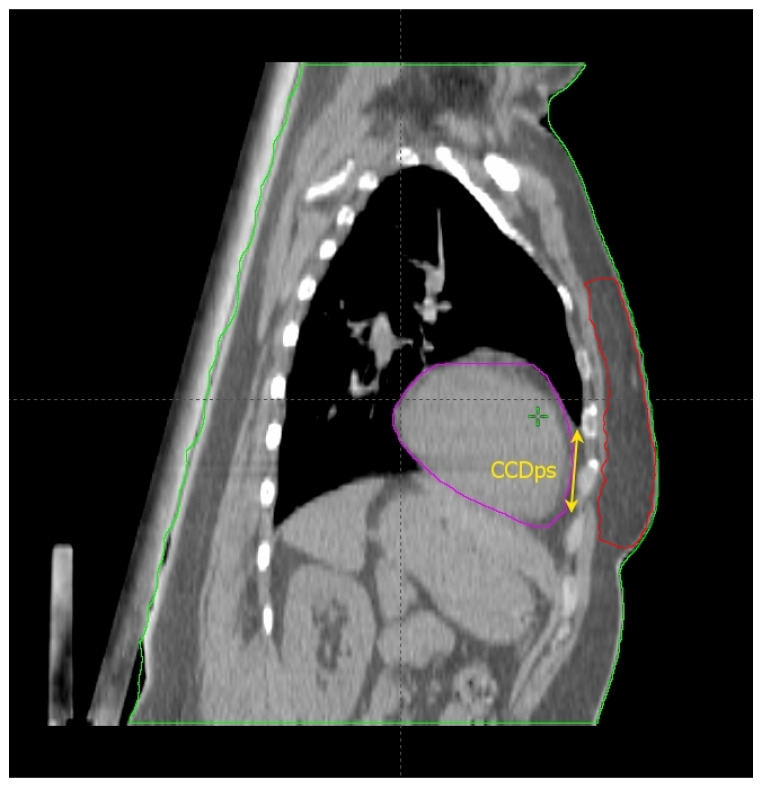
Anatomical parameters on the parasagittal CT plane, chosen as described in the text.
